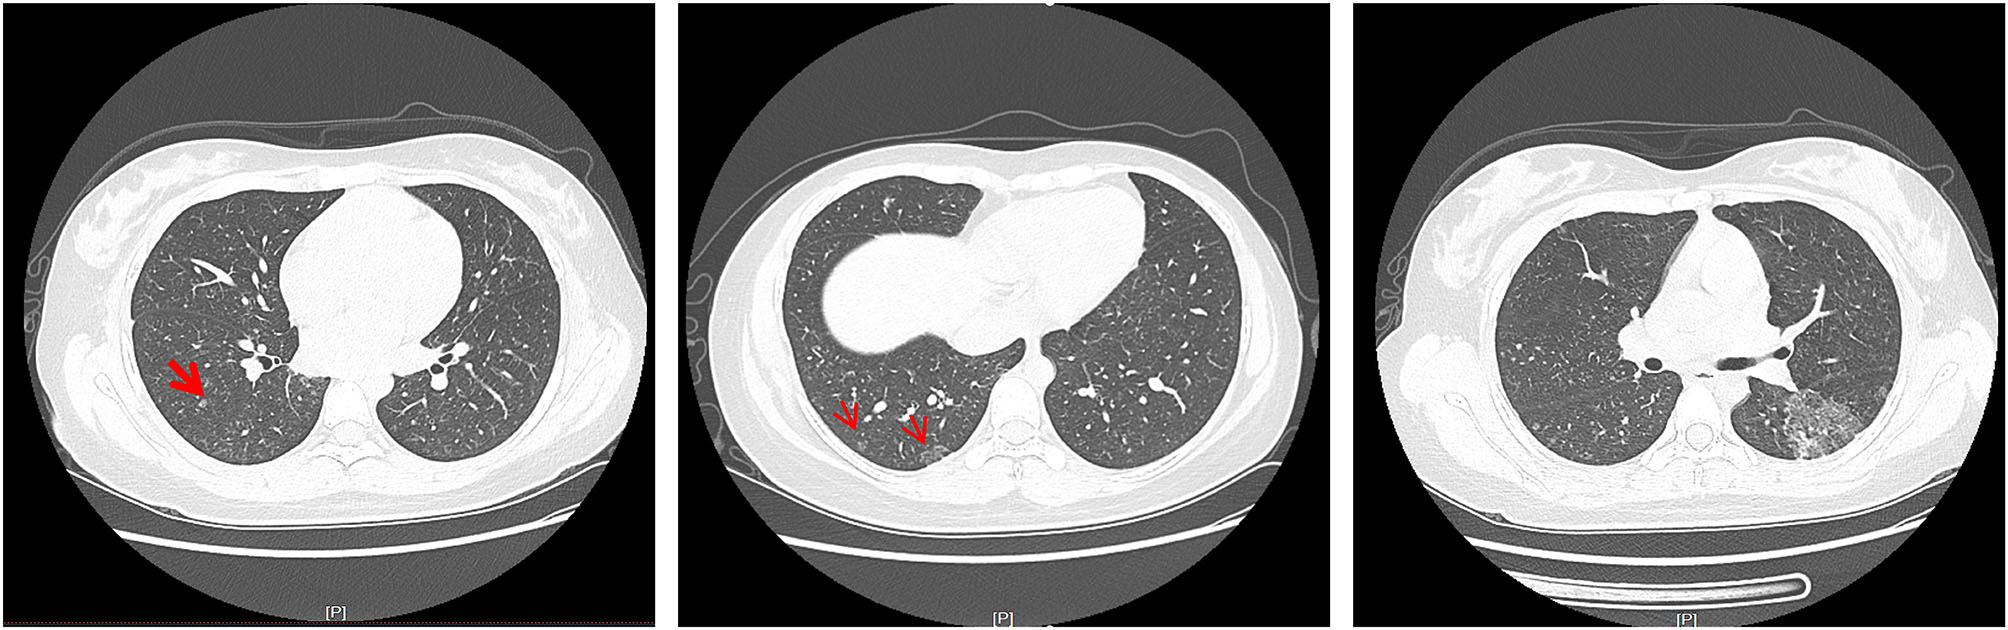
Chest CT examination: speckled, nodular, and flaky high-density shadows in the bilateral lungs
